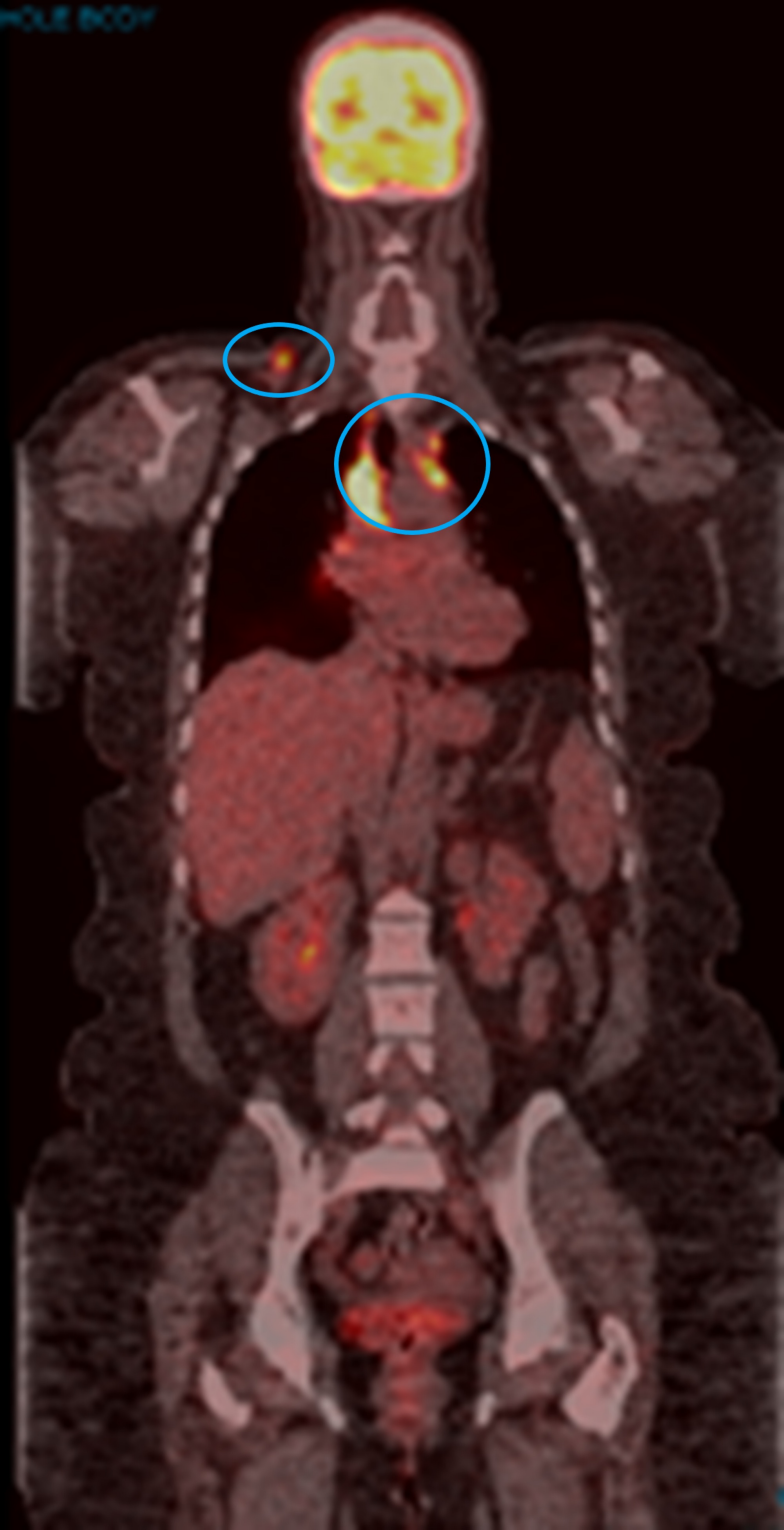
PET/CT scan in February 2017 The image shows FDG-avid lymphadenopathy in the right cervical, supraclavicular, mediastinal, and bilateral hilar regions (blue circles) PET: positron emission tomography; CT: computed tomography; FDG: fluorodeoxyglucose
